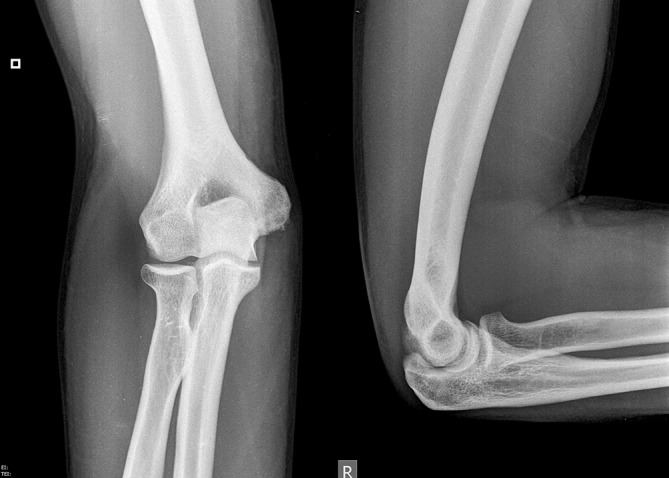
Plain radiographic anteroposterior and lateral views of the elbow, showing calcification of the medial epicondyle as an indirect sign of chronic instability. (Courtesy of the MoRe Foundation)
